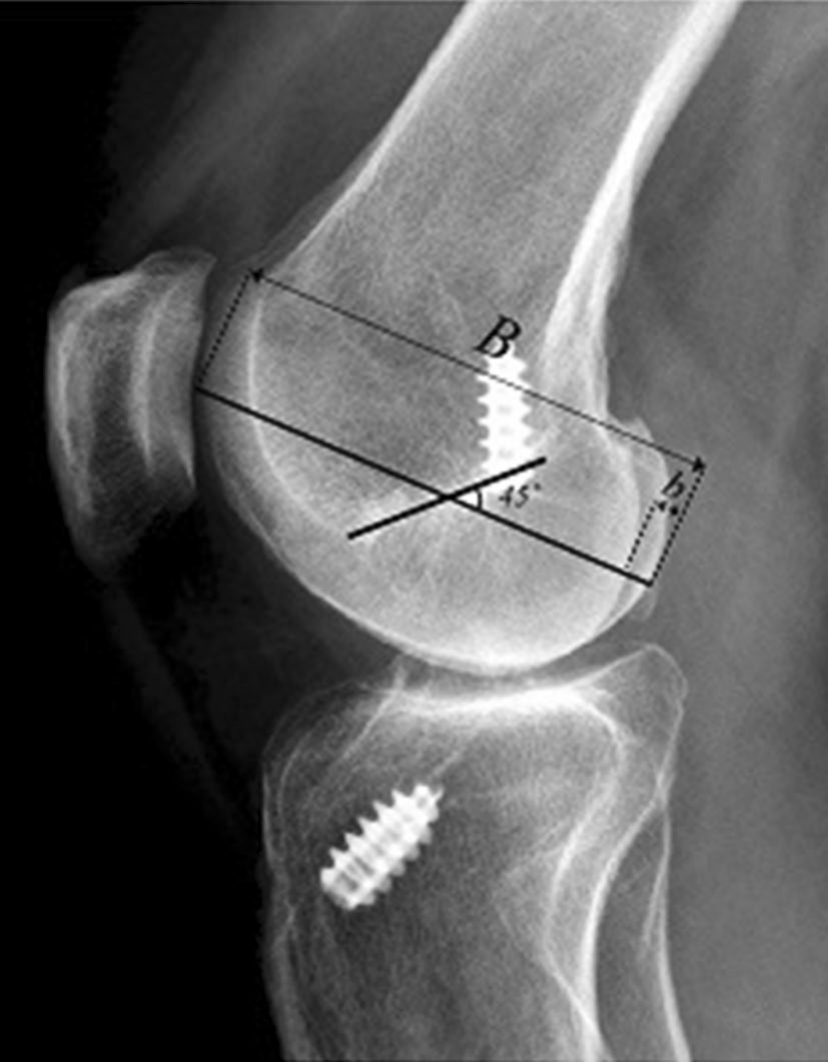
The radiograph shows the newly developed femoral condyle overlap method, used to validate rotation for lateral radiographs. A line was drawn from the posterior to the anterior edge of the femoral condyle (B), intersecting the midpoint at a 45° angle. The angle size was determined as 45°, since it was estimated to represent the anteroposterior axis of the femoral condyle. Moreover, a line (b) was drawn from the posterior limit of the femoral condyle to the border of the femoral condyle situated just anteriorly (corresponding to the medial or lateral condyle depending on the direction of the rotation of the knee), whereafter the femoral condyle overlap can be calculated using an equation
